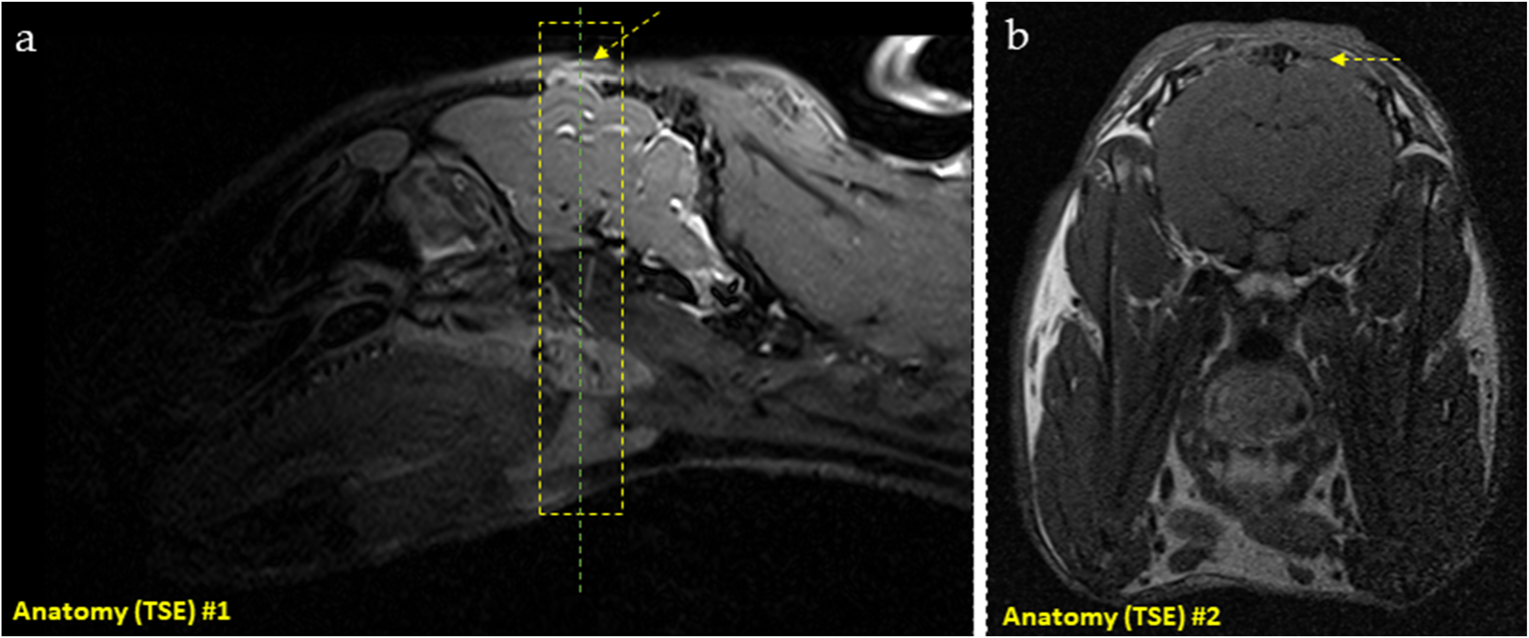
a Sagittal slice of high-resolution anatomical image delineating one of the surgical sites. The yellow rectangle represents the transversal slab used for planning the DCE-MRI slices. b Coronal slice of a high-resolution anatomical image used to confirm localization. This slice corresponds to the dashed line at the center of the rectangle in a. The yellow arrow identifies a specific point that is shown in two different planes
